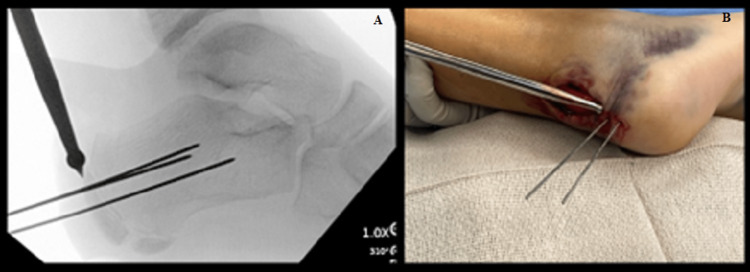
Provisional reduction being held with 2 K-Wires. (A) Lateral radiograph showing placement of two K-wires. (B) Image of the right heel showing two K-wires in place. K-wires, Kirschner wires
